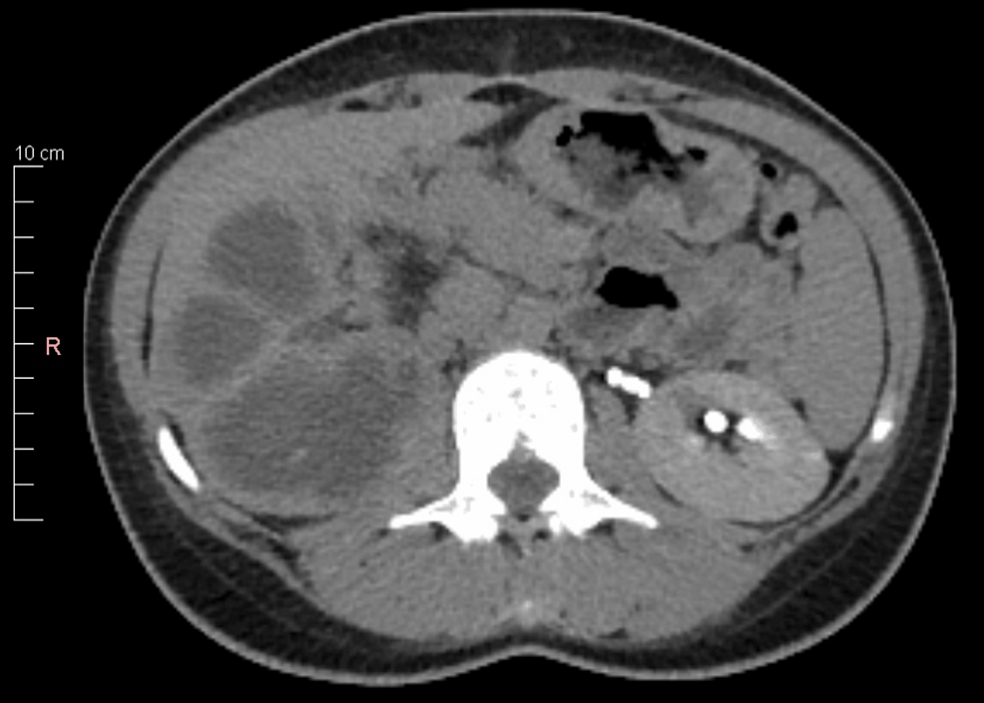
Contrast-enhanced CT image showing right hydronephrosis and non-functional kidney CT: computed tomography
